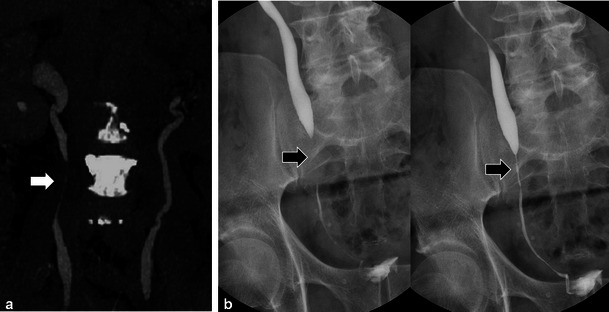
Coronal maximum intensity projection (MIP) from CECT urogram (a) of a 68-year-old man with diffuse metastatic prostate carcinoma (note sclerotic metastases in spine) treated with external beam RT for pelvic disease remotely. Moderate hydroureteronephrosis is present in the right collecting system with a smooth tapered long segment narrowing of the mid right ureter (*white arrow*) in keeping with a radiation induced ureteric stricture. Overhead AP radiographs from antegrade urogram (b) through indwelling nephrostomy tube (not shown) in a different 65-year-old man with previous history of colonic carcinoma treated with low anterior resection and pelvic RT depict a classic radiation induced stricture (*black arrows*) with smooth, tapered margins and long segment involvement just above the uretero-vesicle junction
